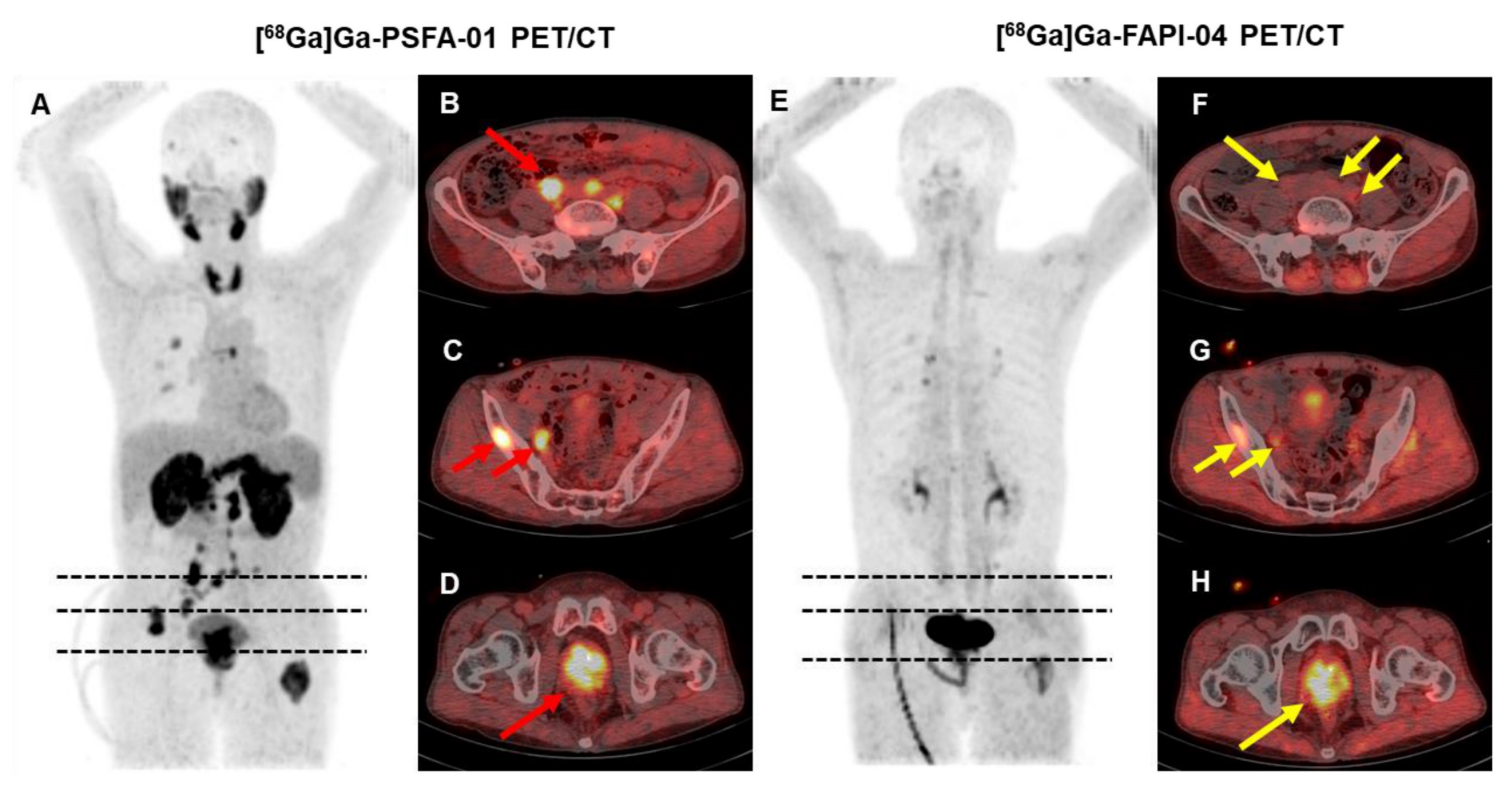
A 66-year-old man (Patient #18) with a Gleason score of 4+3 and a current serum tPSA level of 263.0 ng/mL. [^68^Ga]Ga-PSFA-01 PET/CT imaging revealed the presence of positive lesions in bilateral para-iliac artery and right obturator region (A-D, red arrows), which were not as distinctly evident on [^68^Ga]Ga-FAPI-04 PET/CT (E-H, yellow arrows).
